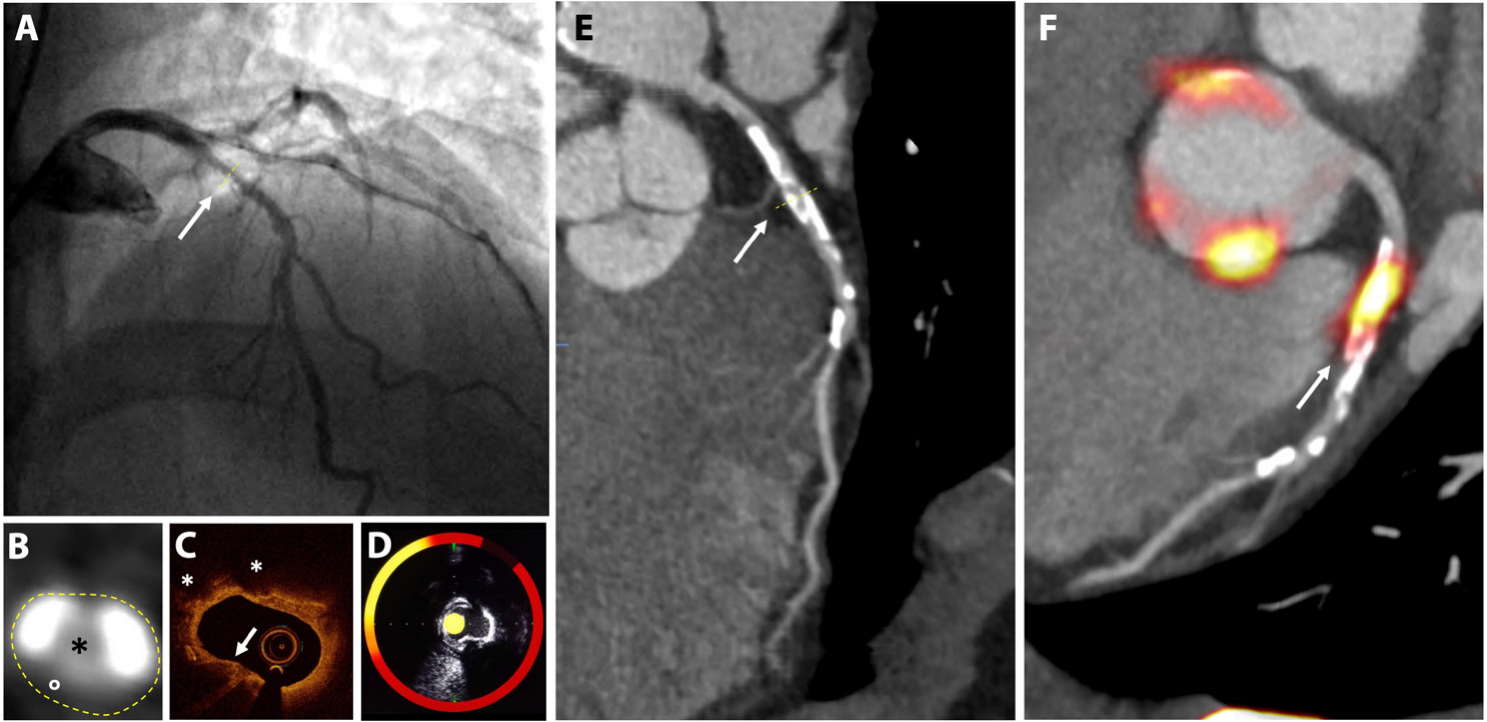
Imaging of coronary atherosclerosis in a patient with non-ST elevated myocardial infarction. (A) Catheter angiography shows an irregular lesion in the proximal left anterior descending coronary artery (artery). (B) Transaxial view of the coronary lesion on CT (i) shows a complex plaque with calcified (white) and fibrofatty (°) plaque around a central lumen (*). (C) Optical coherence tomography detects a thin fibrous cap (arrow) and lipid pools (*). (D) Combined near infrared spectroscopy and intravascular ultrasound confirms high lipid burden within the plaque (yellow). (E) Centreline reconstruction of the left anterior descending artery visualizes calcification and plaque formation throughout the entire vessel. (F) ^18^F-Sodium fluoride positron emission tomography/ CT detects high uptake in the atherosclerotic plaque
